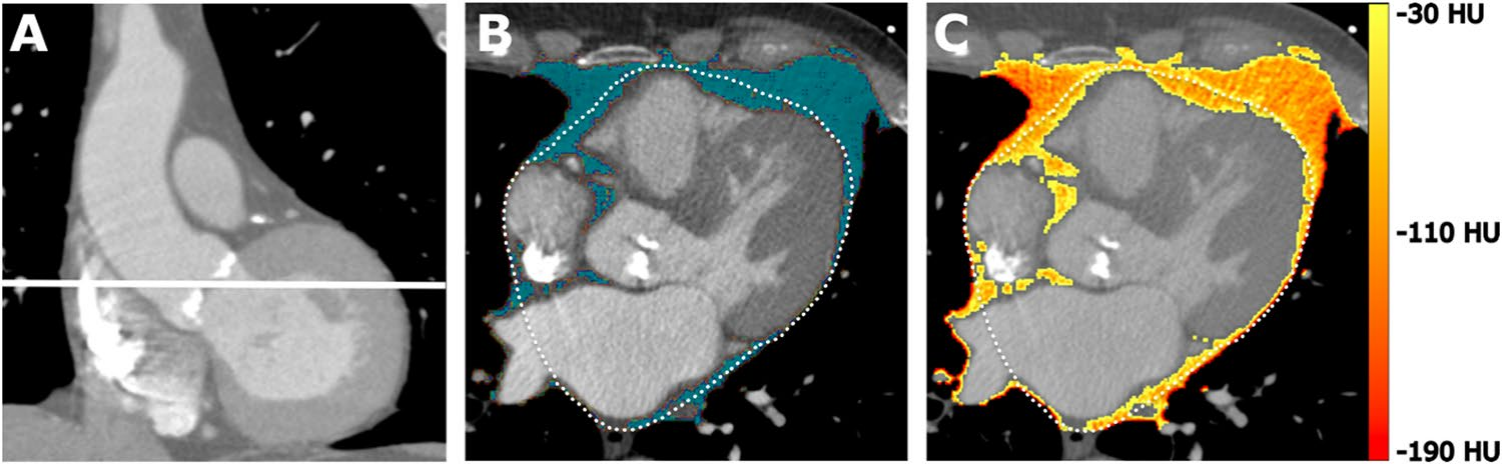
Synopsis of cardiac adipose tissue analysis. Single-slice cross sectional CT images at the level of the aortic valve were exported for analysis (A). Cardiac adipose tissue area was assessed within these cross-sections based on manual delineation of the pericardium and applying densitometric thresholds (B). Mean attenuation of the cardiac adipose tissue was calculated to obtain the inflammatory activity of the adipose tissue (C). HU = Hounsfield Units.
